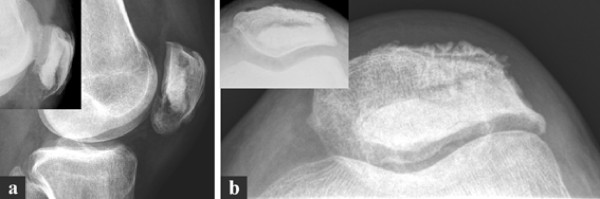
Radiographs of the left patella after bone cement packing. Radiographs of the patella 17 months after surgery. The small X-rays were performed 1 week after filling of the cavity with bone cement. Note the lack of the inferior cortex of the patella (a - small), which is remodelled at last follow-up. b shows degenerative changes at last follow-up but without clinical symptoms.
